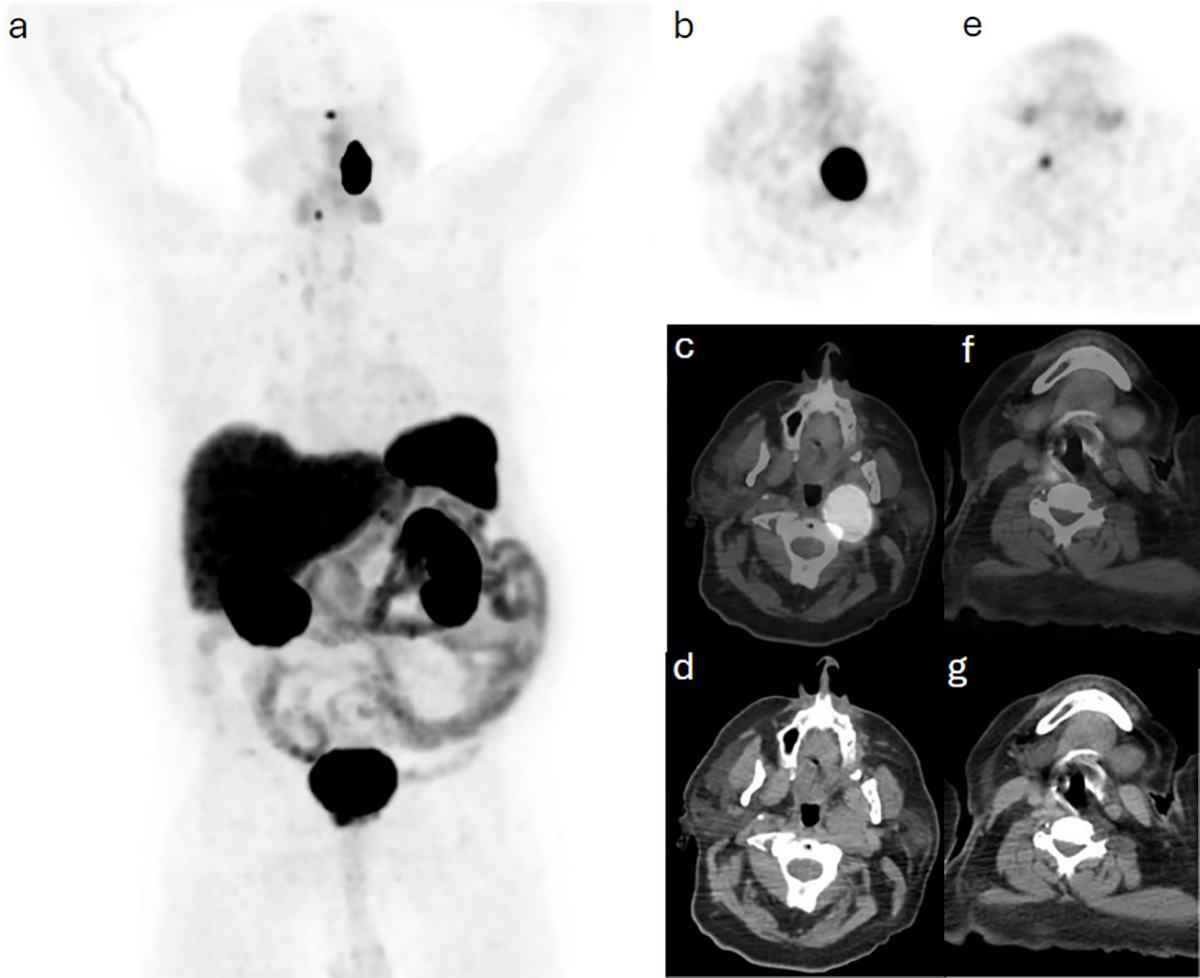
Case 1. (a) maximum intensity projection (MIP) demonstrating intense Ga68-DOTA-TATE uptake in the head and neck region bilaterally, which are further confined to a soft tissue lesion in the left retropharyngeal space and a smaller right focal tissue on trans-axial slices including (b, e) PET, (c, f) hybrid PET/CT and (d, g) CT images.
